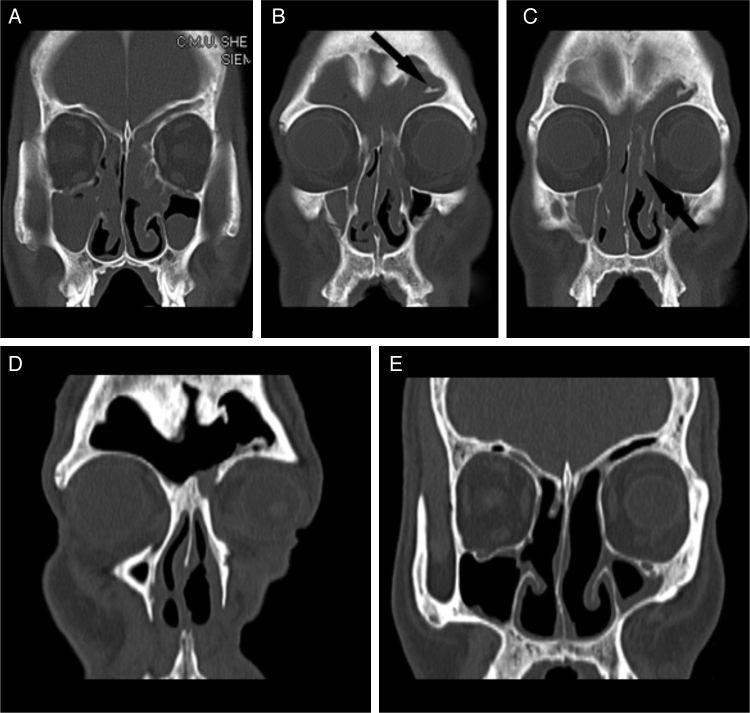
(A–C) Preoperative coronal computed tomography (CT) scan showing: (A) a hyperdense mass occupying both nasal cavities and the bilateral frontal, bilateral ethmoid, and bilateral maxillary sinuses; (B) the well-pneumatized frontal sinus, destruction of the frontal sinus septum, a bone septum on the lateral wall of the left frontal sinus (black arrow), and a slight deviation of the nasal septum to the right; and (C) the enlarged frontal ostium and focal hyperostosis in the left ethmoid sinus (black arrow). (D and E) Postoperative coronal CT scan after complete removal of the mass. There was no sign of recurrence two years after surgery.
